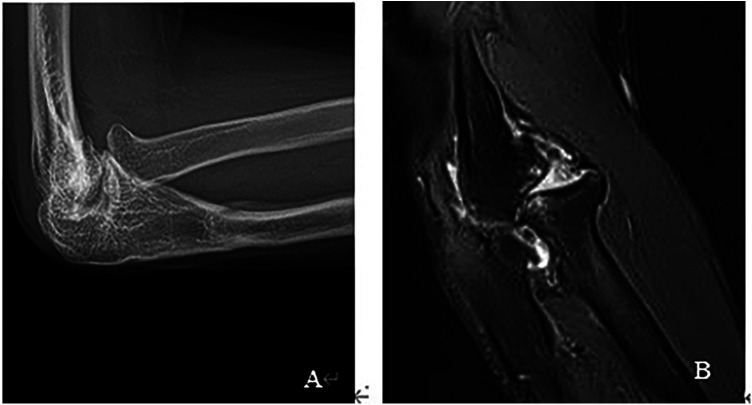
(A) 1 case subluxation and osteoarthritis in x-ray, (B) 1 case subluxation and osteoarthritis in MRI.
